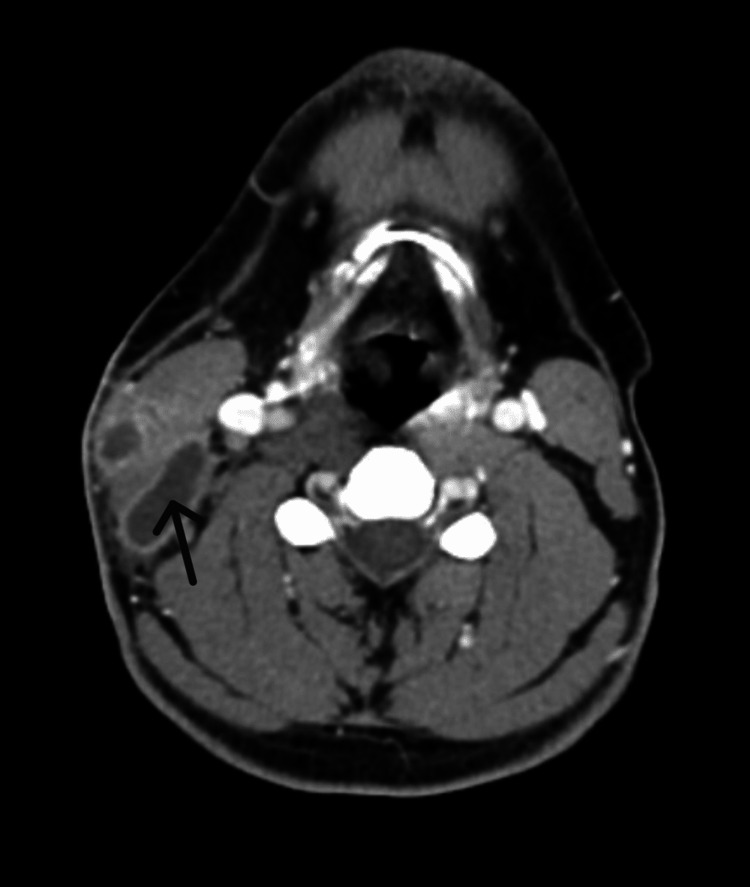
CT soft tissue neck with IV contrast
